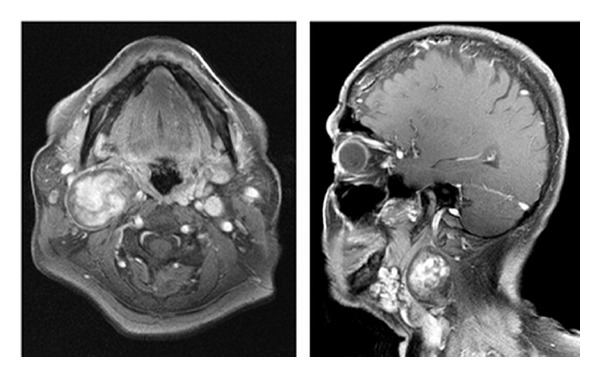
Postcontrast MRI of the neck demonstrating a mass at the level of the carotid bifurcation.
